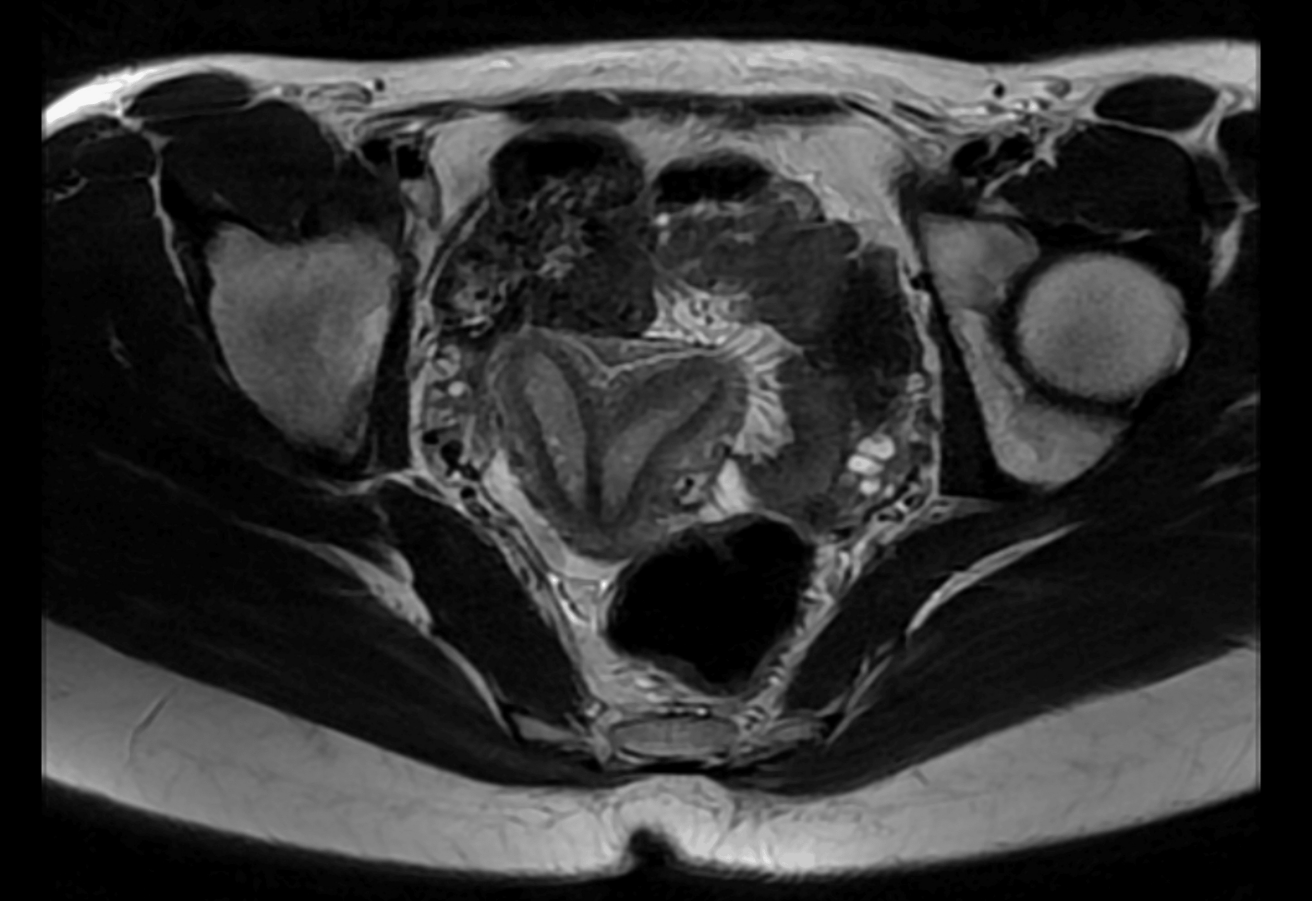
Complete septate uterus (MRI) MRI: magnetic resonance imaging
